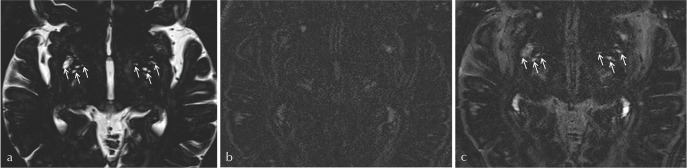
A 71-year-old woman with suspected endolymphatic hydrops. On magnetic resonance cisternography (MRC) (a), there is much perivascular space (PVS) in the bilateral basal ganglia (arrows). On pre-contrast heavily T_2_-weighted FLAIR (hT_2_-FL) image, the PVS has a low-signal intensity (b). On post-contrast hT_2_-FL images (c), the PVS has an increased signal intensity (arrows). The cerebrospinal fluid (CSF) also has increased signal. Note that some of the PVS has a higher signal than the CSF.
